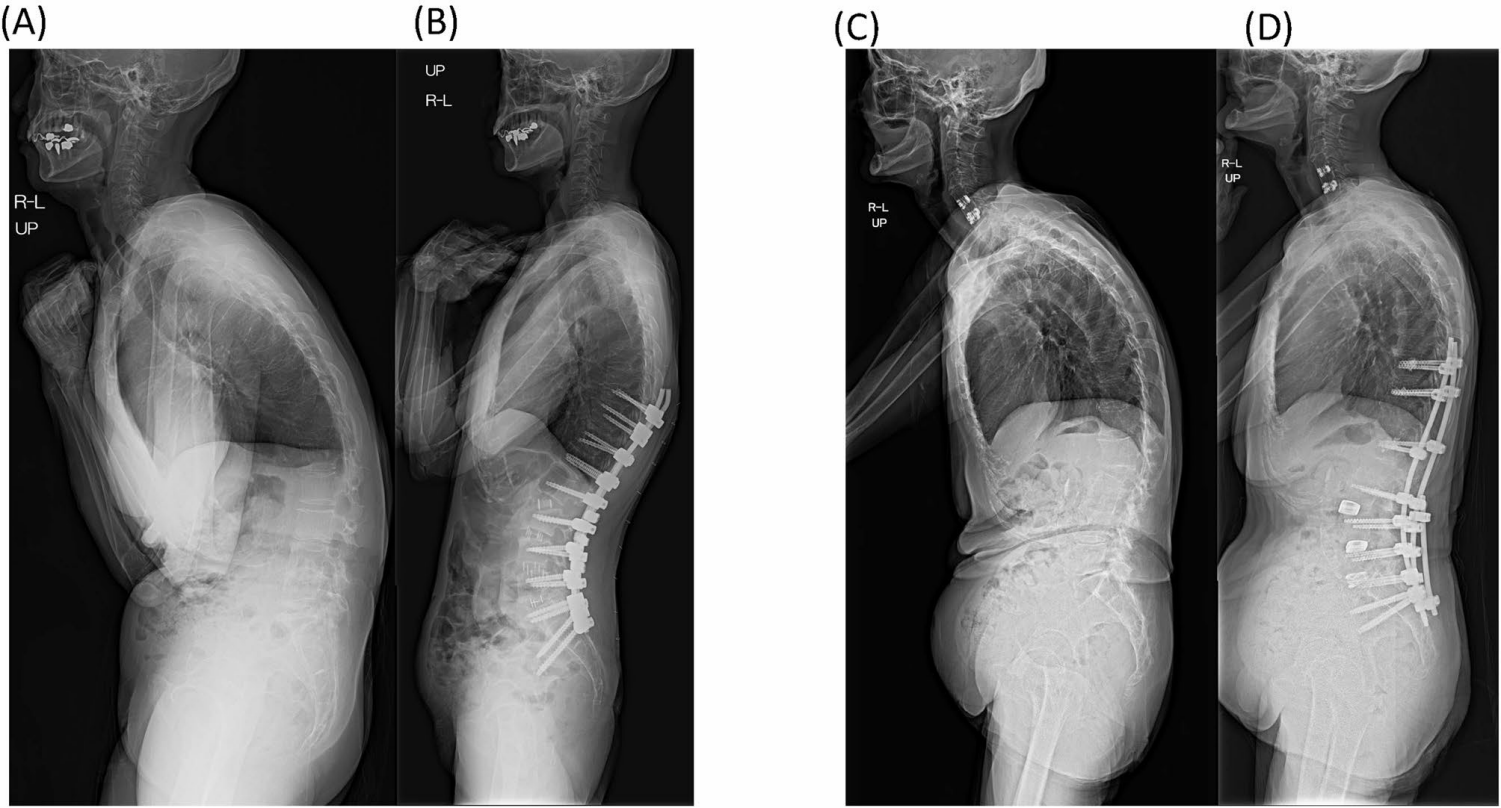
Representative Radiographs from PJF and Non-PJF Cases. This figure shows preoperative and postoperative standing lateral radiographs for one patient who developed PJF and one who did not. (A) Preoperative radiograph of a patient who developed PJF. (B) Postoperative radiograph of the same patient. (C) Preoperative radiograph of a patient who did not develop PJF. (D) Postoperative radiograph of the same patient.
